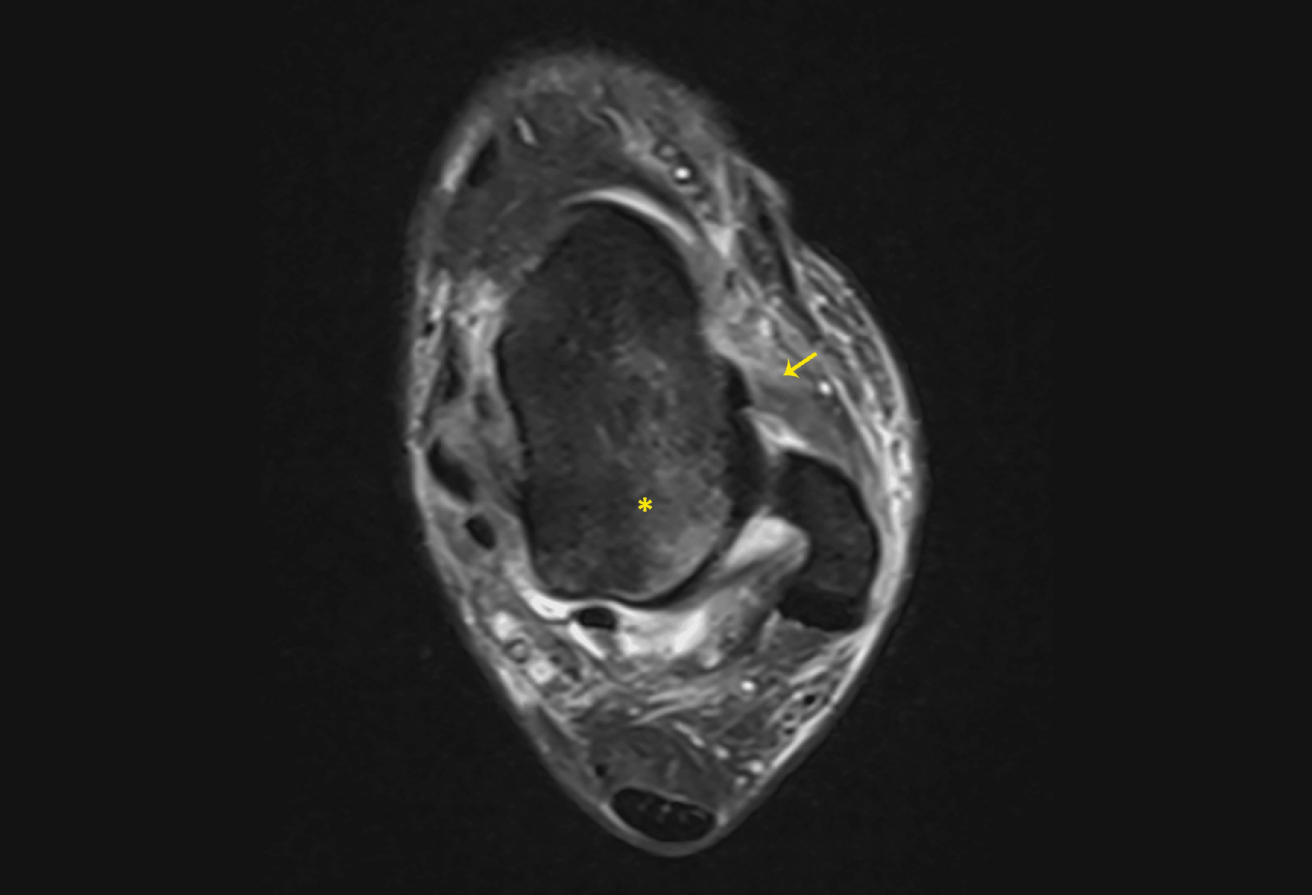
Axial proton density fat-saturated MRI of the ankle demonstrating soft tissue and bone injuries. The anterior talofibular ligament appears thickened and partially disrupted, consistent with a sprain (yellow arrow). Associated bone contusion edema is visible in the adjacent talus (yellow asterisk).
